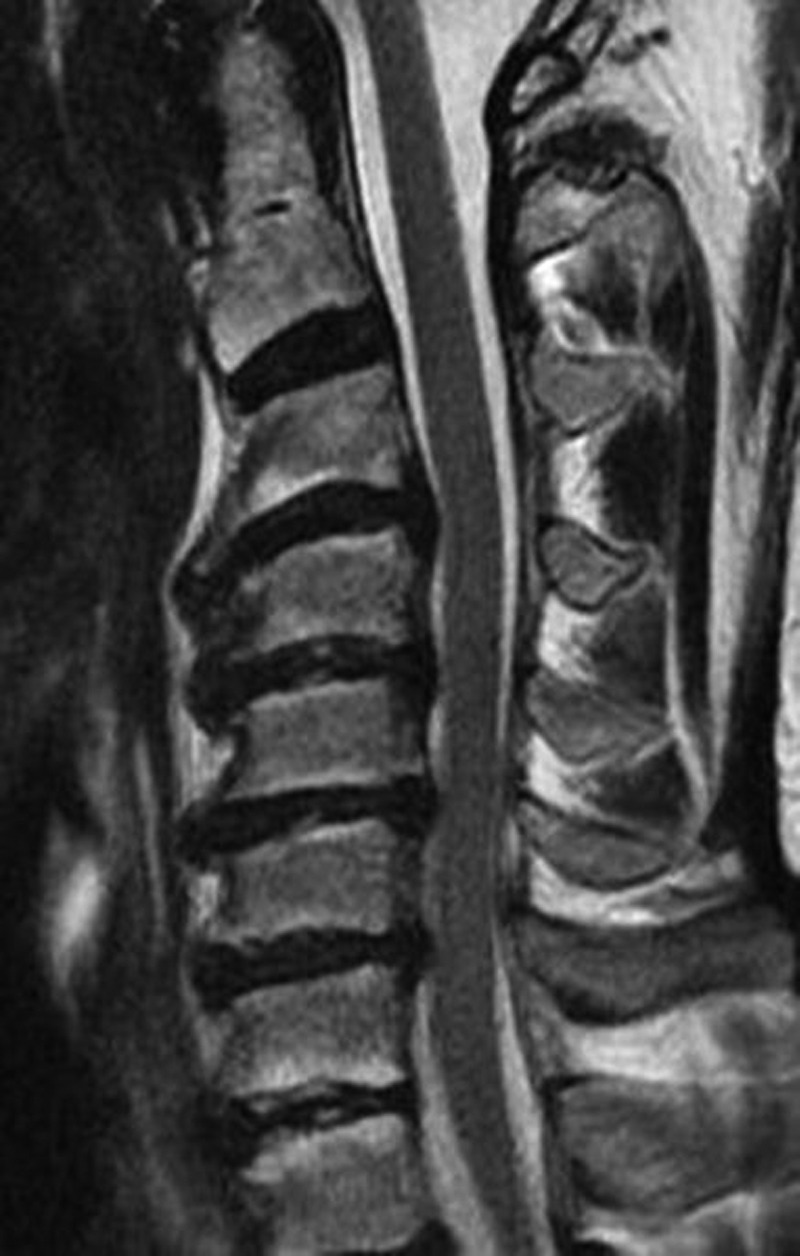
Case Example of a Four-Level ACDF Using the Optio-C Implant A 65-year-old man presented with progressive cervical spondylotic myelopathy with a sagittal T2 weighted MRI demonstrating four levels of cervical stenosis.
